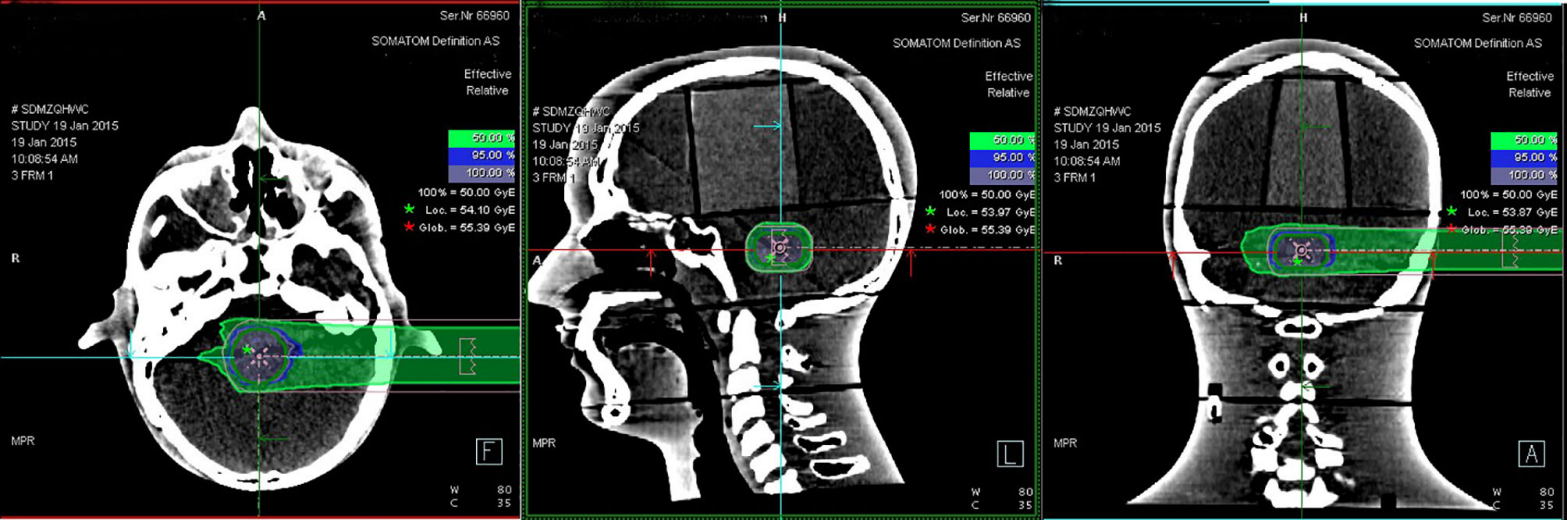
Example of rigid registration fusion for planned CT and PET/CT image.
